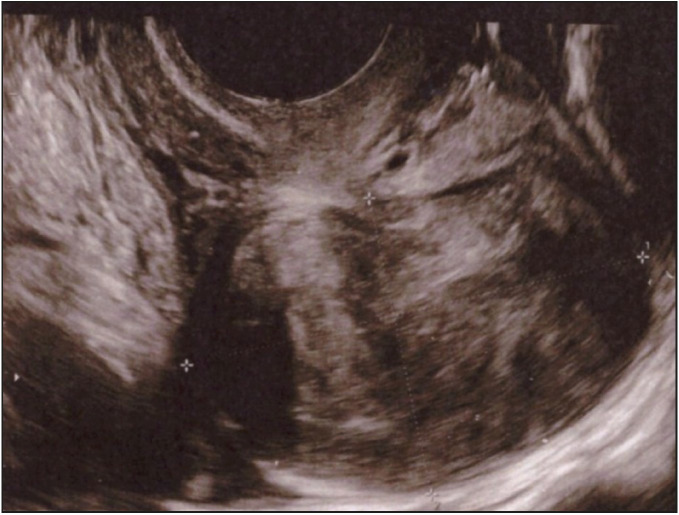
Transvaginal ultrasound shows a well-defined, solid subserosal mass, with variable echogenicity, that causes acoustic shadowing, suggestive for leiomyoma.
